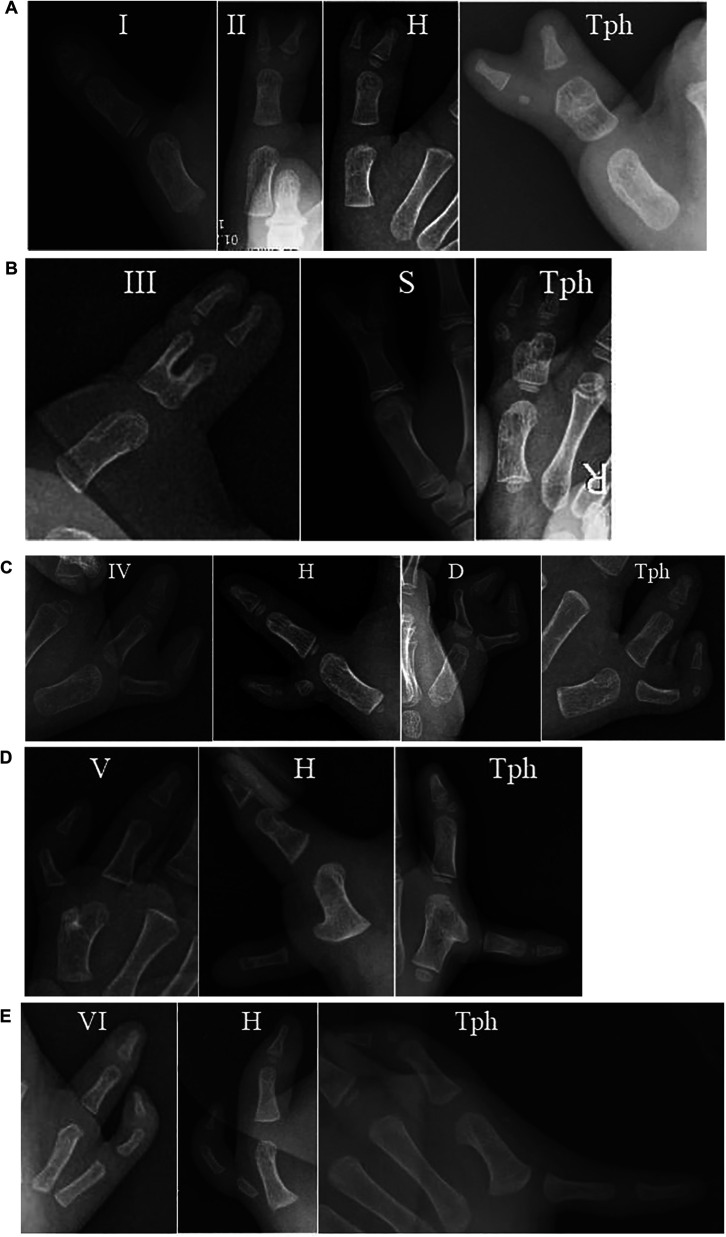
(A–E) Radiograph of the Rotterdam Classification. (A) type I, II-II, II-H and II-Tph; (B) type III-III, III-S and III-Tph; (C) type IV-IV, IV-H, IV-D and IV-Tph; (D) type V-V, V-H and V-Tph; (E) type VI-VI, VI-H and VI-Tph. H, hypoplastic or floating; D, deviation; Tph, triphalangism; S, symphalangism.
